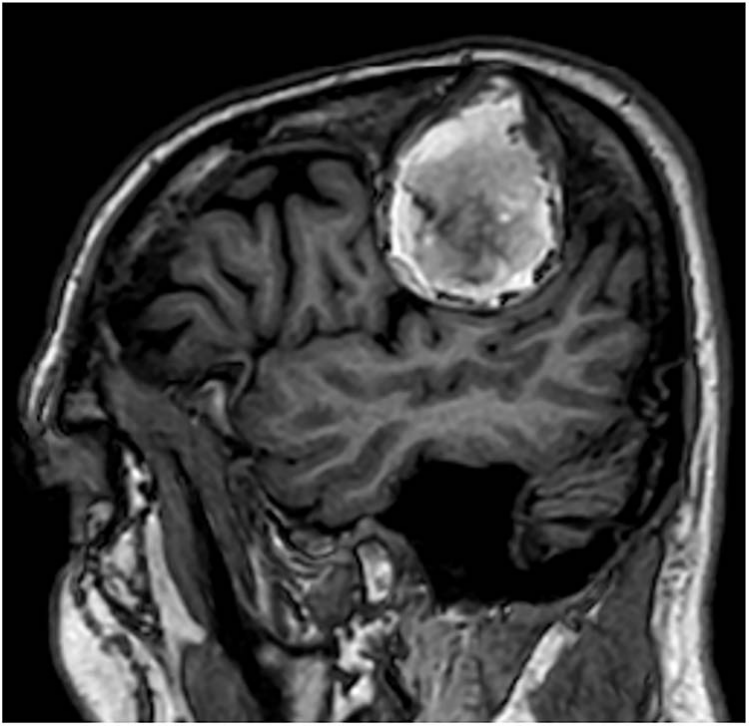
T1-weighted contrast-enhanced sagittal MRI scan showing the lesion with intralesional haemorrhagic products. There is no evidence of dural invasion.
